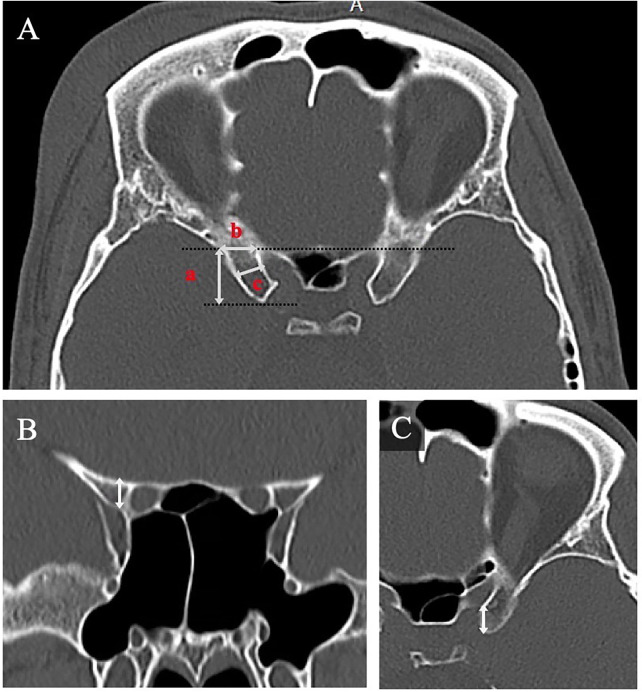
Morphometric measurements of the anterior clinoid process (ACP) and examples of Type 0 and Type 1 pneumatization (A) axial CT image demonstrating right ACP length (a), base width (b), and midpoint width (c) (B) coronal CT image demonstrating ACP base thickness (arrow). The right ACP shows no pneumatization (Type 0), while the left ACP demonstrates pneumatization limited to the optic strut (Type 1). (C) axial CT image demonstrating ACP tip-to-optic strut distance (arrow).
